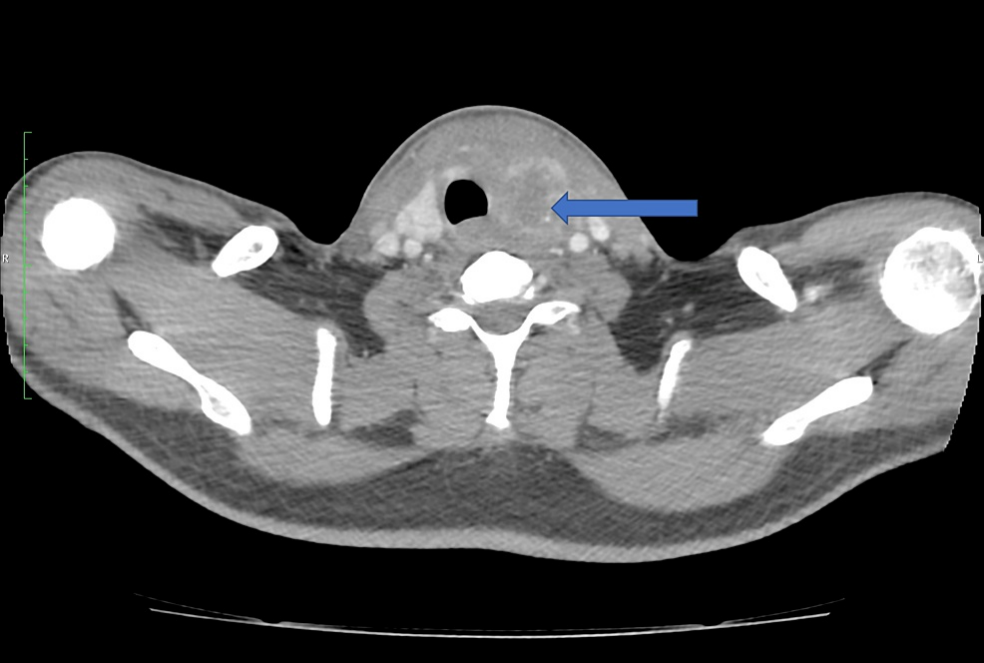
CECT image of the neck Contrast-enhanced computed tomography (CECT) neck showing thyroiditis with nodule and a large ill-defined hypo-dense lesion in the left lobe of the thyroid, extending to the isthmus, suggestive of an evolving abscess within the thyroid nodule (shown in blue arrow).
